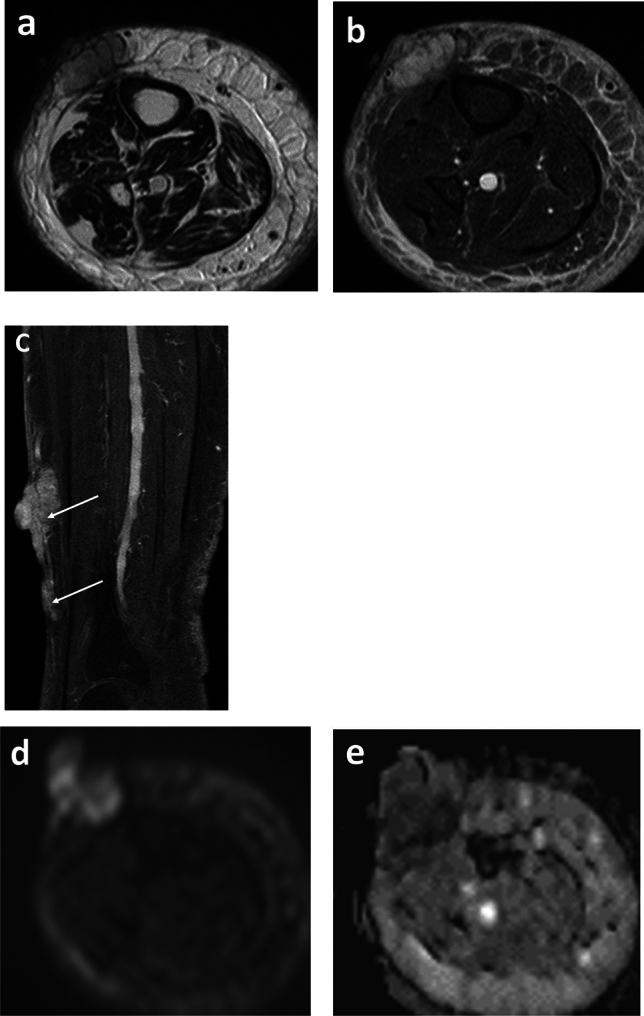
A 64-year-old female with an 8-year history of lower extremity disuse edema caused by cerebral infarction, presented with hemorrhagic masses of the left lower leg (case 2). a and b Axial T2-weighted and fat-suppressed T2-weighted images show an ill-defined mass with a confluent multinodular shape. c On sagittal fat-suppressed T2-weighted image, multiple masses in close proximity are identified (arrows). d and e DW image and ADC map show a hyperintense mass with low ADC values (ADC_mean_ = 0.83 × 10^−3^ mm^2^/s).
